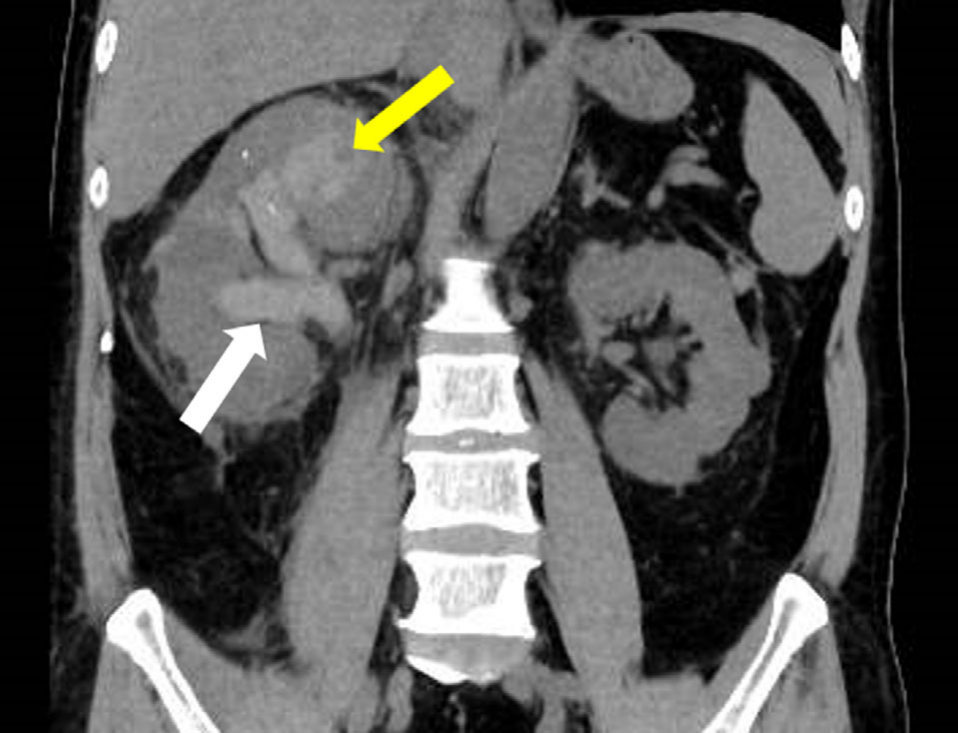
Non‐contrast CT on day 8 demonstrates a hematoma extending from the right renal pelvis to the ureter (white arrow). A high‐density area was observed within the renal parenchyma near the superior calyx (yellow arrow). There was a clear change when compared to the simple CT at admission.
